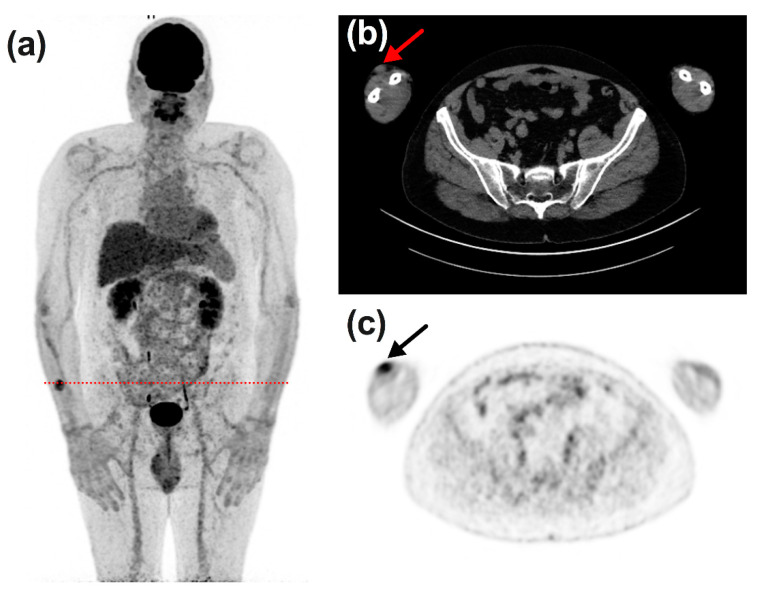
65-year-old male patient diagnosed with stage IV trunk melanoma (Breslow 0.7 mm, BRAF+) and treated with nivolumab. ^18^F-FDG PET/CT—(a), maximum intensity view; (b) CT transverse slice; (c) PET transverse slice} depicted progression of a subcutaneous nodule after 20 cycles of treatment, while ceCT determined stable disease. This patient was treated by radiotherapy.
